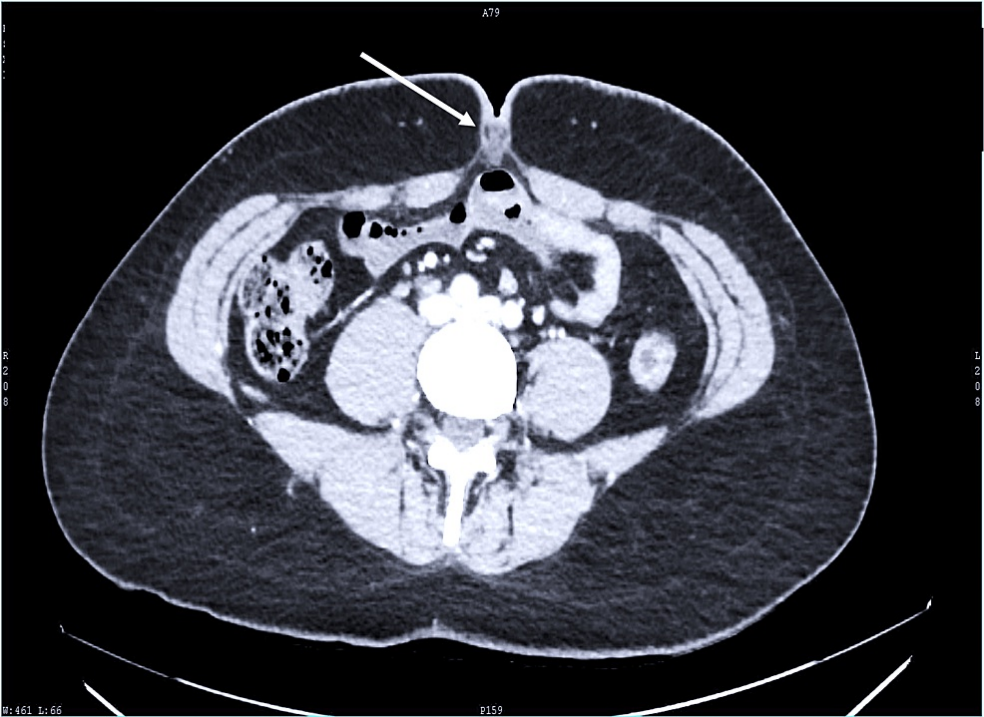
CT scan axial view showing the UC as depicted by the white arrow. UC, urachal cyst
